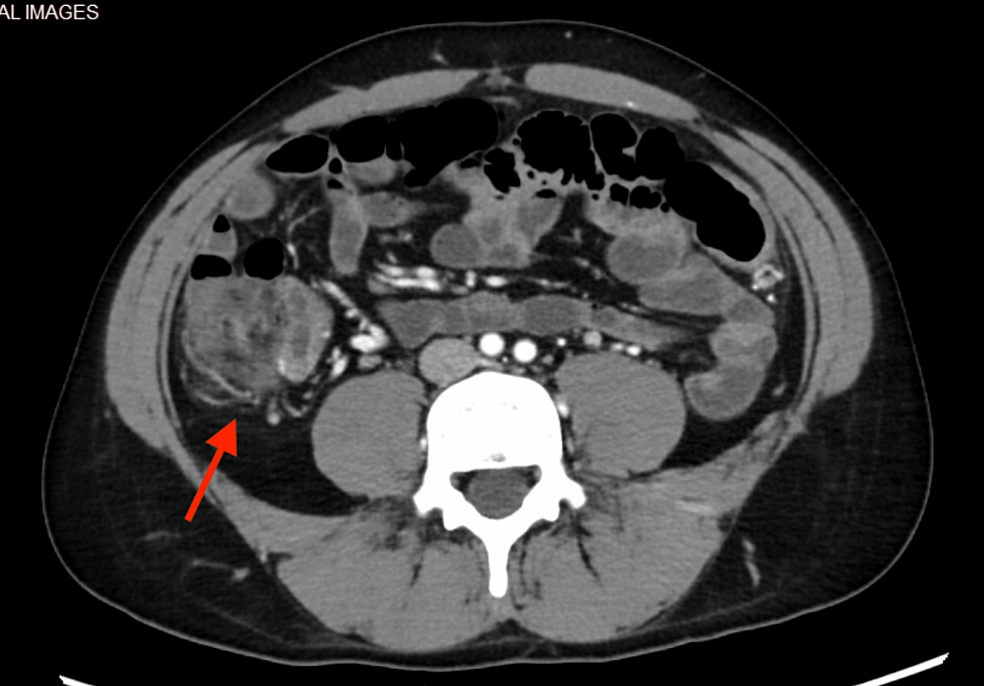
Luminal hyperemia of terminal ileum A computed tomography (CT) enterography with contrast revealed mild luminal hyperemia of the terminal ileum.
